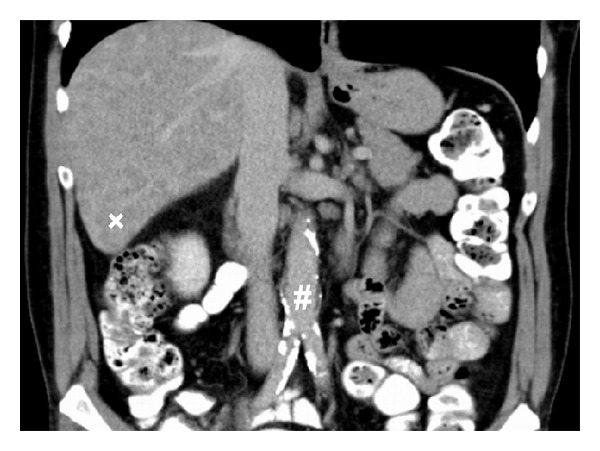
Abdominal CT-scan showing diffuse calcifications of the infrarenal aorta and aortic bifurcation (#) as well as mild ectasia (3 cm). Note the diffuse hepatic metastatic disease (x).
